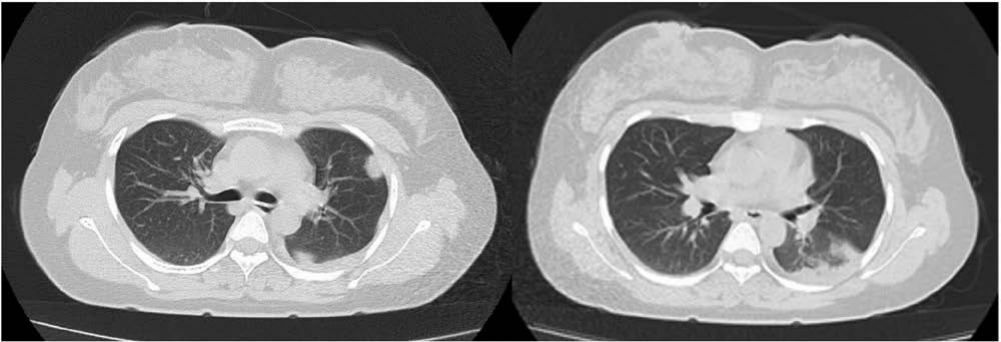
Three weeks later, 16-slice computerized tomography reexamination images of the chest revealed reexpansion of the shadows on the CT images after antibiotic and methylprednisolone withdrawal.
